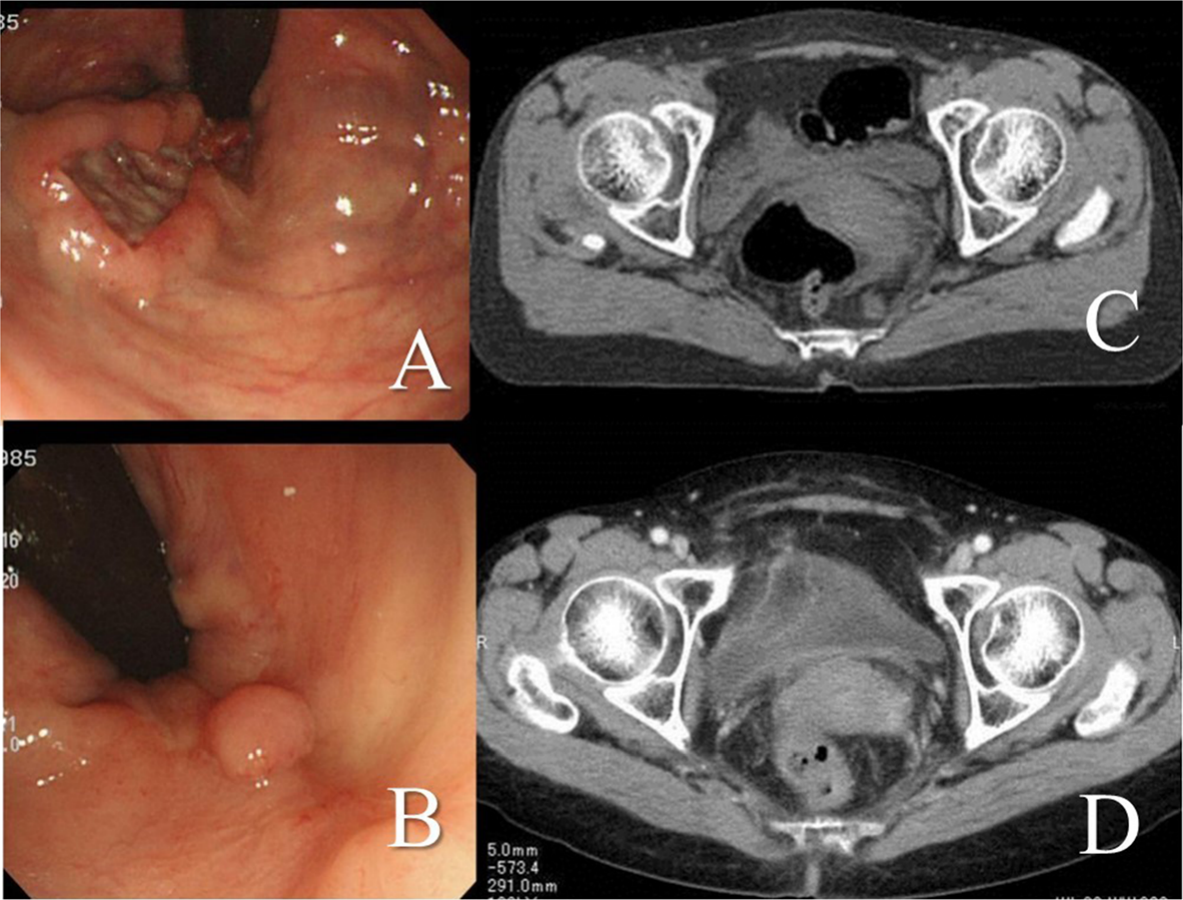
Effectiveness of chemoradiation therapy with S-1 for patient 1 evaluated by colonoscopy and computed tomography. Colonoscopy was showing an ulcerative lesion in the lower rectum (A). Lymph node metastasis in the mesorectum was suspected using computed tomography (C). After chemoradiation therapy with S-1, colonoscopy showed a polypoid lesion (B). Biopsies revealed that there were no tumor cells, and lymph node metastasis had disappeared (D).
